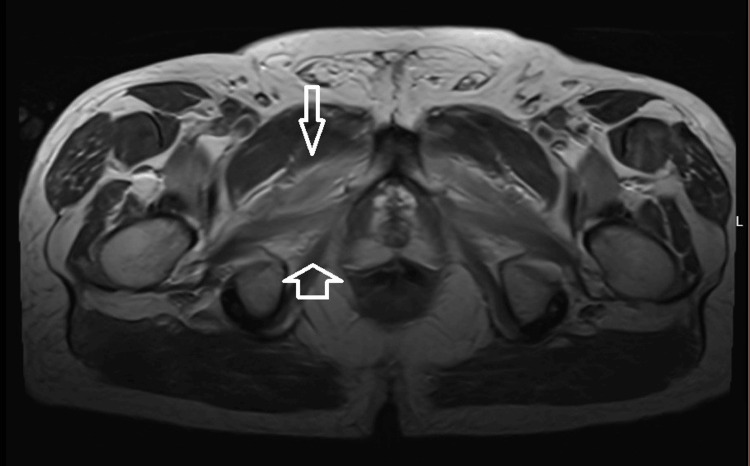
Axial T2 MRI Highlighting Symmetric Hyperintensities in the Hip Flexor Muscles and Adductor Muscles Consistent With Inflammatory Myopathy Long arrow (top): Pointing to the hip flexor muscles demonstrating the increased T2 signal consistent with myositis. Short arrow (bottom): Pointing to the adductor muscles demonstrating T2 hyperintensity.
